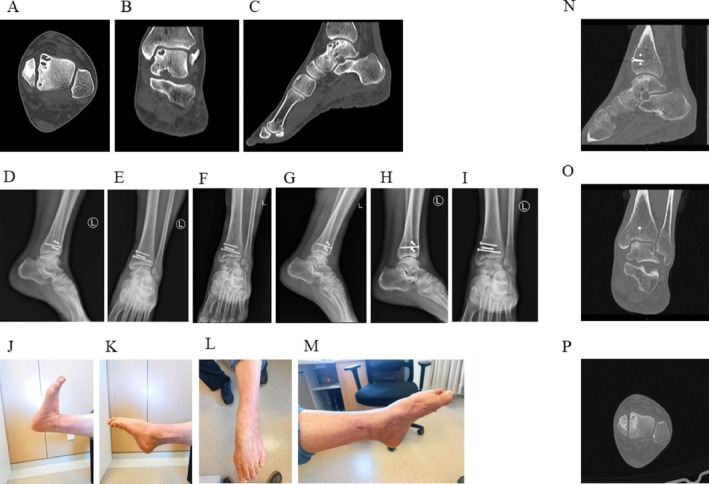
Images from another typical case of a large talar cyst. (A–C) Preoperative computed tomography (CT) images demonstrating a cystic lesion in the left talus—axial view (A), coronal view (B), and sagittal view (C); (D and E) lateral (D) and anteroposterior (E) radiographs of the left ankle on the second postoperative day showing satisfactory osteotomy reduction and screw placement; (F and G) radiographs at 3 months postoperatively showing indistinct osteotomy lines and early graft incorporation; (H and I) radiographs at 4 months postoperatively demonstrating progressive graft assimilation; (J–M) clinical photographs at 1 year postoperatively showing well‐healed surgical incisions and good functional recovery—dorsiflexion, lateral view (J), plantarflexion, lateral view (K), plantarflexion, frontal view (L), and plantarflexion, medial view (M); (N–P) Postoperative CT images at 16 months demonstrating complete fusion of the grafted bone with the surrounding talus and no evidence of cyst recurrence or degenerative changes—sagittal view (N), coronal view (O), and axial view (P).
